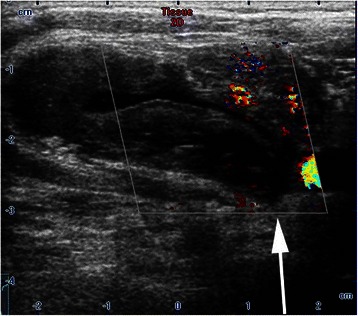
The ultrasound re-scanning showed the right cervical cystic mass without pulsative hemorrhage after embolization.
